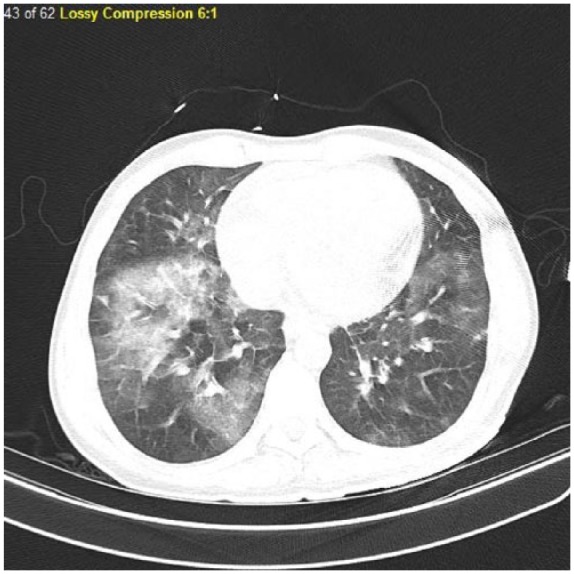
Computed tomography of the chest showing bilateral infiltrates attributed to eosinophilic pneumonia.
